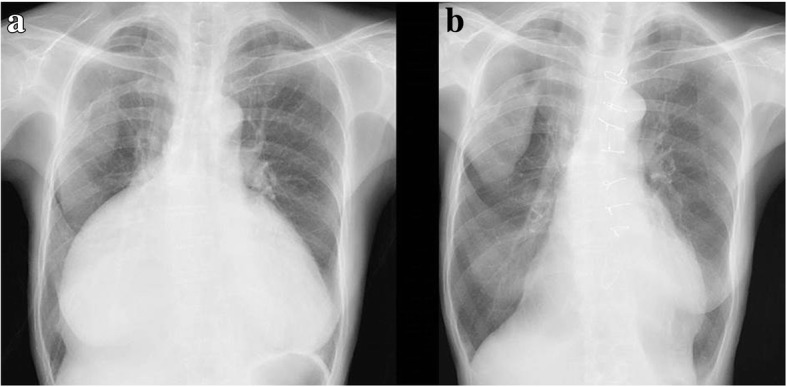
a Chest X-ray at preoperative examination showed severe enlargement of the heart, with a cardiothoracic ratio of 88%. b Postoperative chest X- ray showed reduced right-side shadow of the cardiac silhouette
